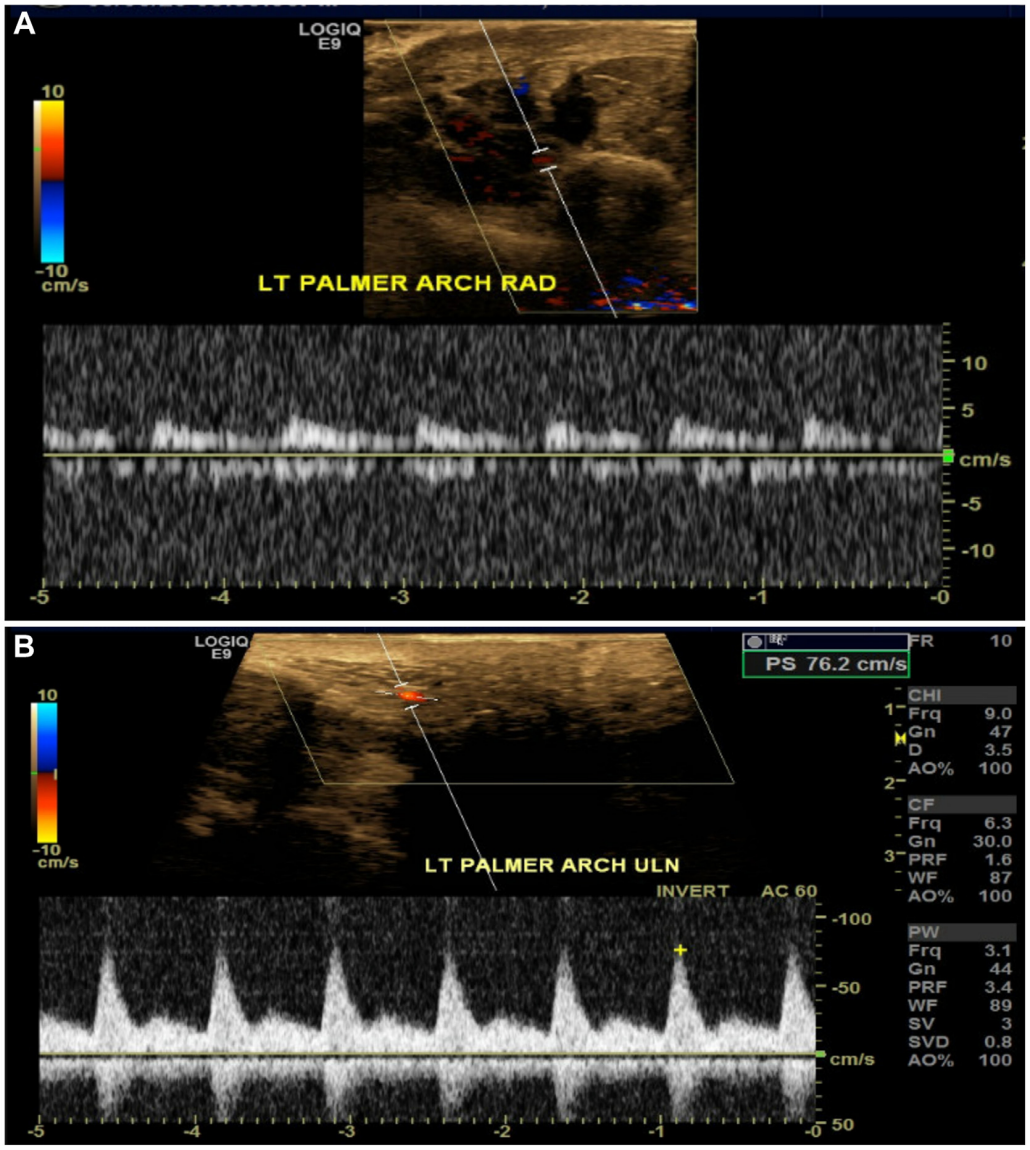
(A) Duplex arterial ultrasound image of the radial side of the palmar arch. Waveform exhibited is monophasic, intermediate resistive. (B) Duplex arterial ultrasound image of the ulnar side of the palmar arch. Waveform exhibited is multiphasic.
